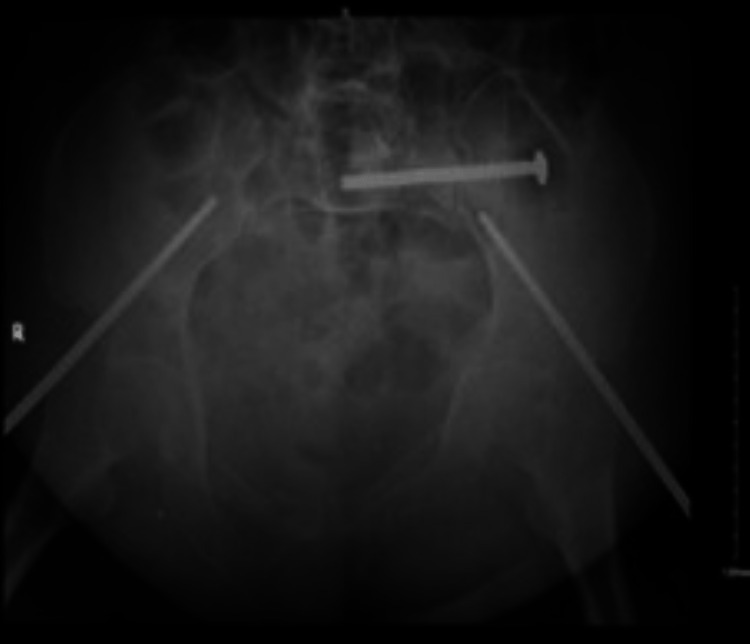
Radiograph demonstrating immediate postoperative anterior external fixator with a left sacroiliac screw.
